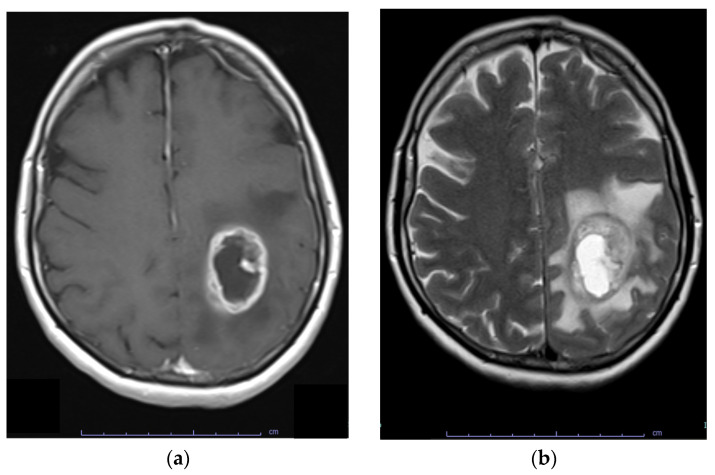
Contrast-enhanced MRI of a glioblastoma. (a) Contrast-enhanced T1 image.; (b) T2 image showing oedema.
